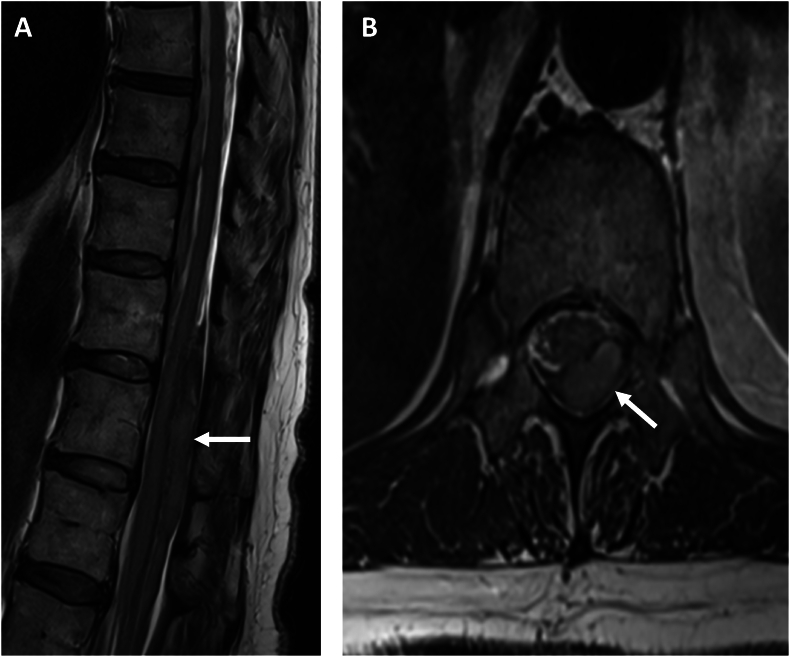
Sagittal (A) and axial (B) T2-weighted MRI showing an intradural spinal hematoma exerting mass effect on the spinal cord (white arrows).
